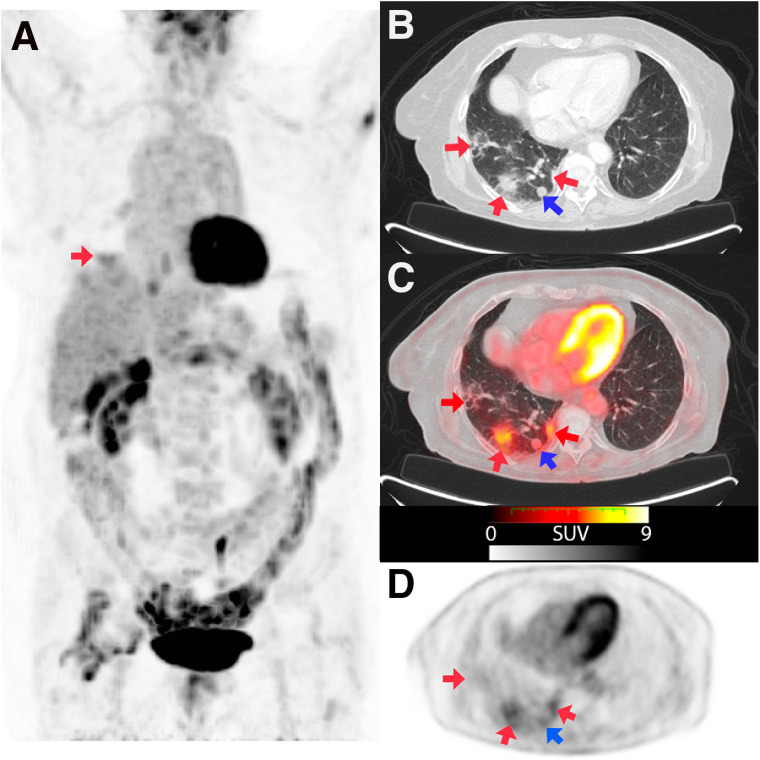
An 86-y-old woman with second primary lung tumor (B and C, blue arrow) referred for PET/CT to assess therapeutic response. Maximum-intensity projection (A) and axial sections with lung window (B) and fusion (C) images showed several pulmonary consolidations located mainly in right inferior lobe (red arrows, CO-RADS 5), with increased ^18^F-FDG uptake (SUV_max_, 4.6). rRT-PCR was positive for COVID-19.
